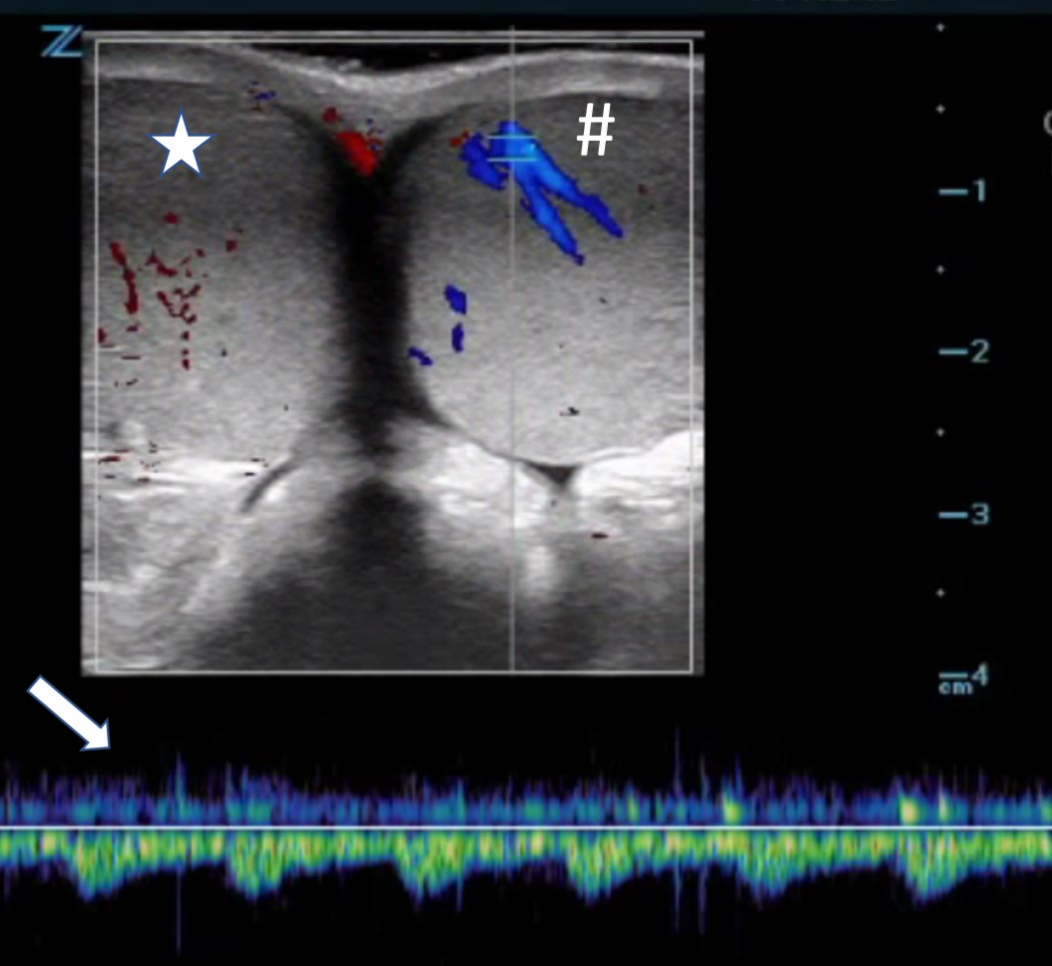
Point-of-care testicular ultrasound using doppler ultrasound imaging - Appropriate flow is demonstrated to the left testicle (#) indicated by color signal and arterial waveform on pulse wave doppler (arrow). No consistent color signal is appreciated over the right testicle (star), confirming the diagnosis of testicular torsion.
